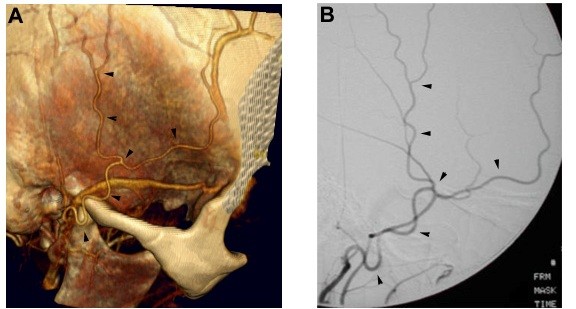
Evaluation of the superficial temporal artery with CTA. Comparison of the right superficial temporal artery (STA) as seen by CTA with surface-shaded display three-dimensional reformation (A), and by conventional invasive catheter angiography (B). Note the similarity of the STA as reproduced on the CTA compared with that seen on invasive catheter angiography. In addition, information with respect to relational anatomy with the skull and adjacent structures can be appreciated with the CTA.
